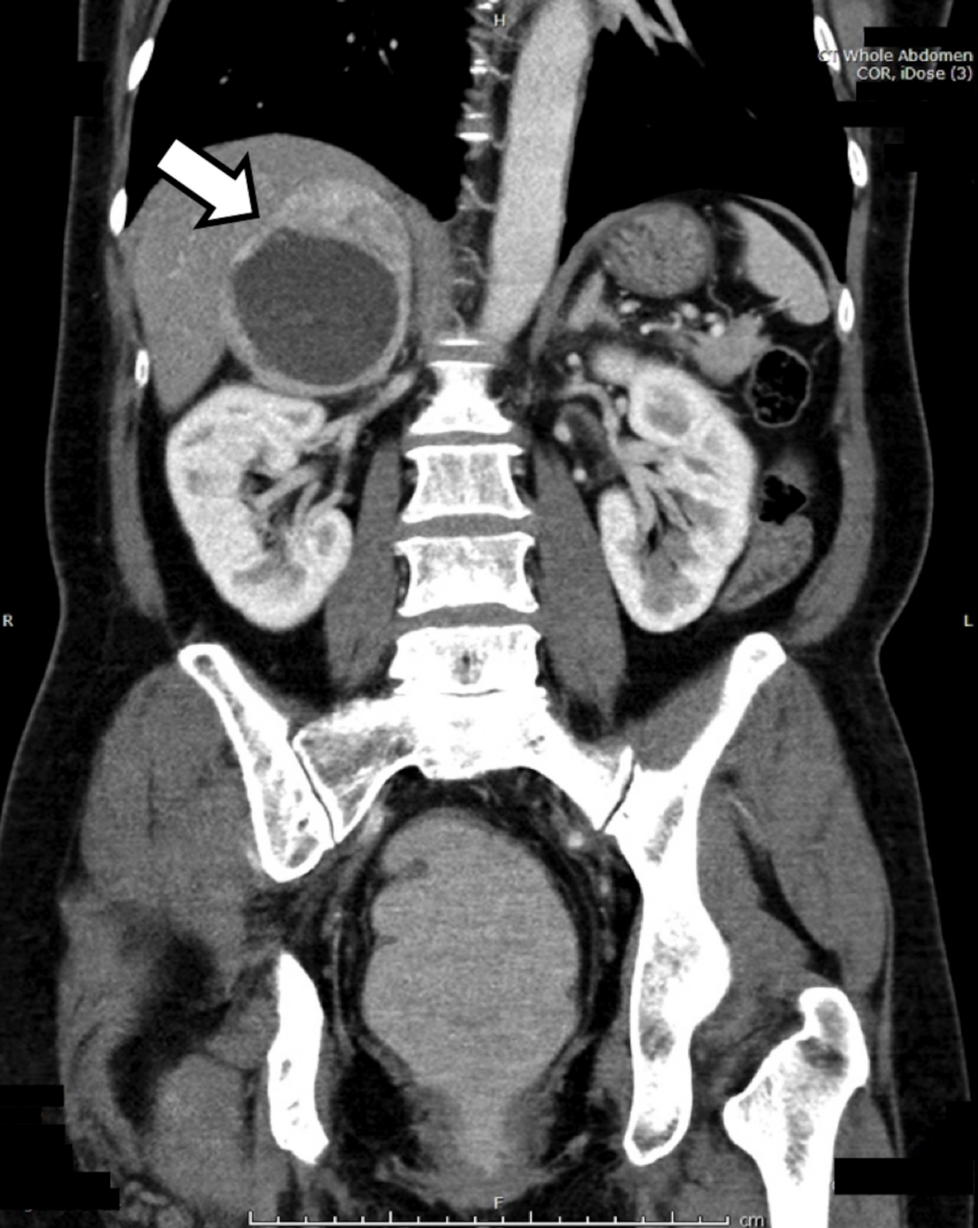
Computed tomography (CT) of the upper abdomen Contrast-enhanced coronal CT image demonstrates a large heterogeneously enhancing complex cystic-solid right adrenal mass.
